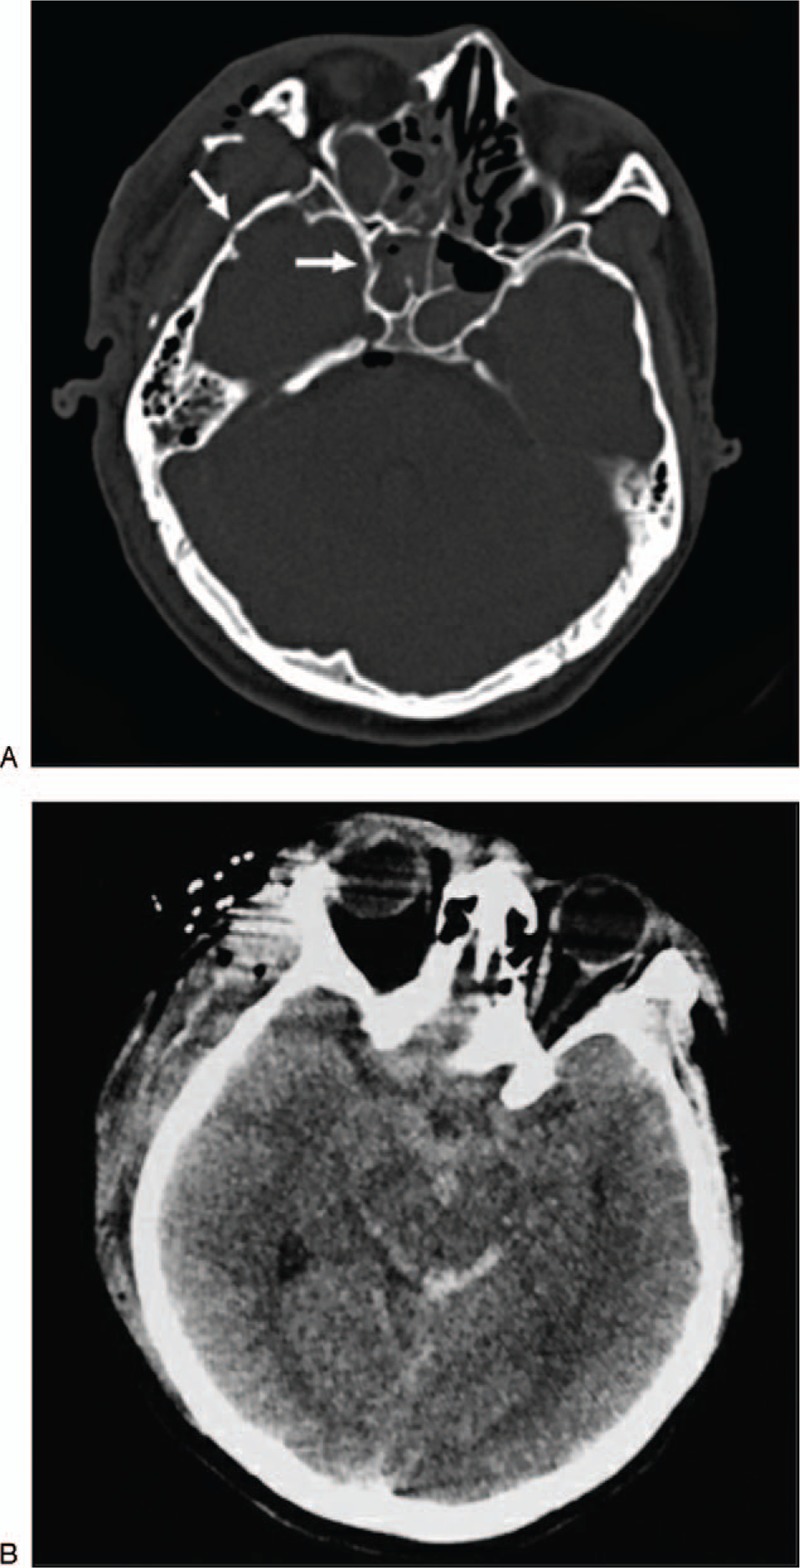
(A and B) Computed tomography on admission shows fracture of the temporal bone (arrow) and sphenoid sinus (arrow) and subtle perimesencephalic subarachnoid hemorrhage.
